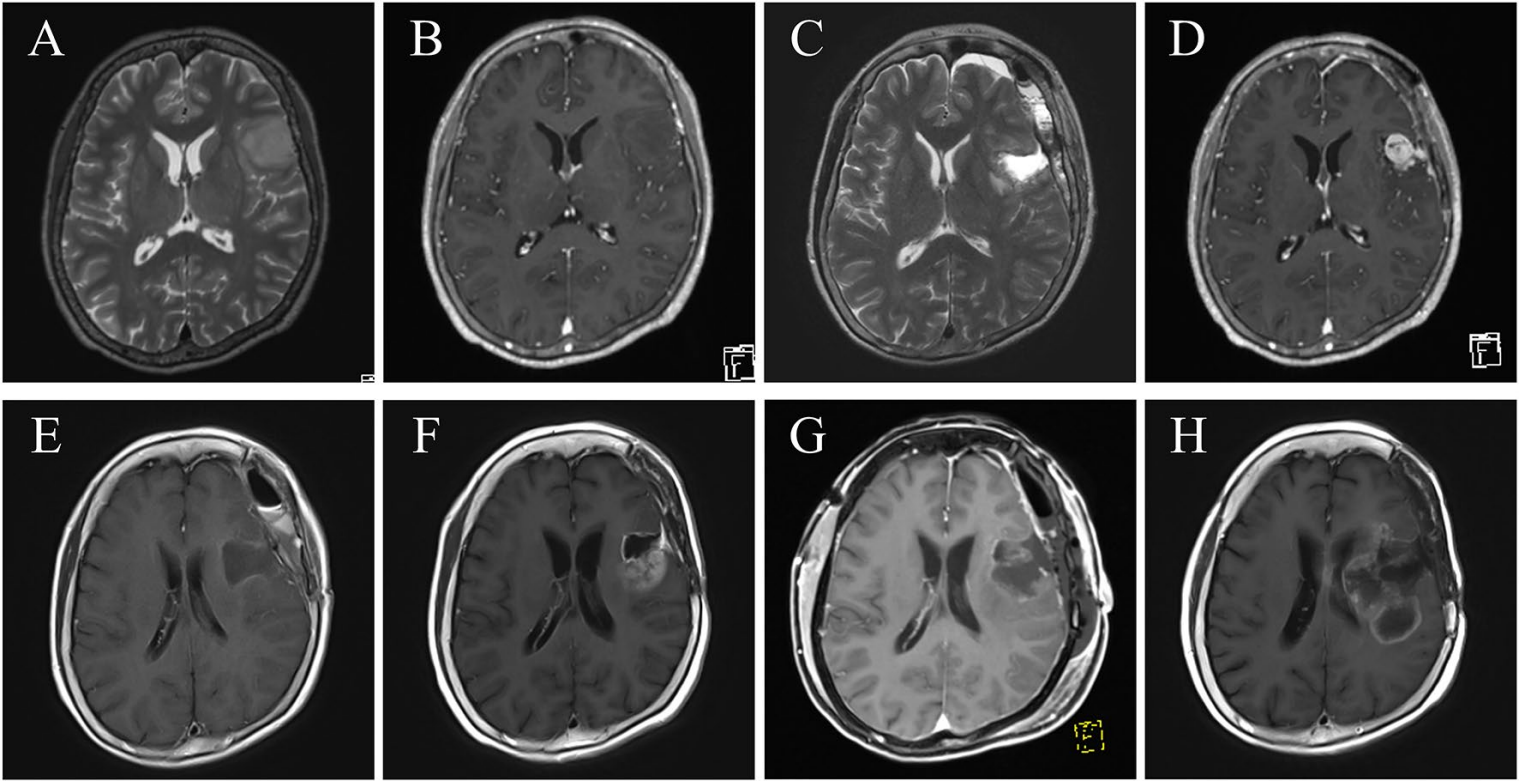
(A) Preoperative axial T2-weighted and (B) axial T1-weighted MRI with gadolinium enhancement, showing a high-intensity area with no enhancement in the left inferior frontal gyrus forming a localized tumor mass. (C) Postoperative axial T2-weighted MRI showing no tumors due to gross-total resection (first surgery). (D) Axial T1-weighted MRI with gadolinium enhancement performed about 6 months after the first surgery, showing nodular enhancement in the left frontal surgical cavity. (E) Postoperative axial T1-weighted MRI with gadolinium enhancement, showing no tumors due to gross-total resection (second surgery). (F) Axial T1-weighted MRI with gadolinium enhancement performed about 4 months after the second surgery, showing enhancing mass lesion in the surgical cavity again. (G) Postoperative axial T1-weighted MRI with gadolinium enhancement, showing no tumors owing to gross-total resection (third surgery). (H) Axial T1-weighted MRI with gadolinium enhancement at last follow-up, showing recurrent tumor. Tumor progression could not be controlled.
